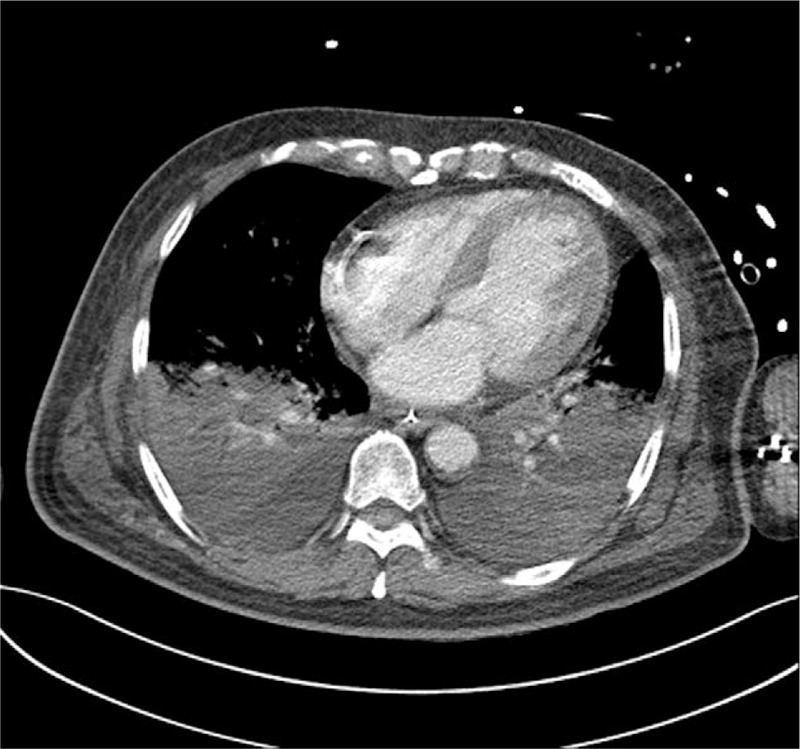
Computed tomography scans showing consolidation (bilateral pleural and fissural effusion) and interlobular septal thickening in both lungs.
